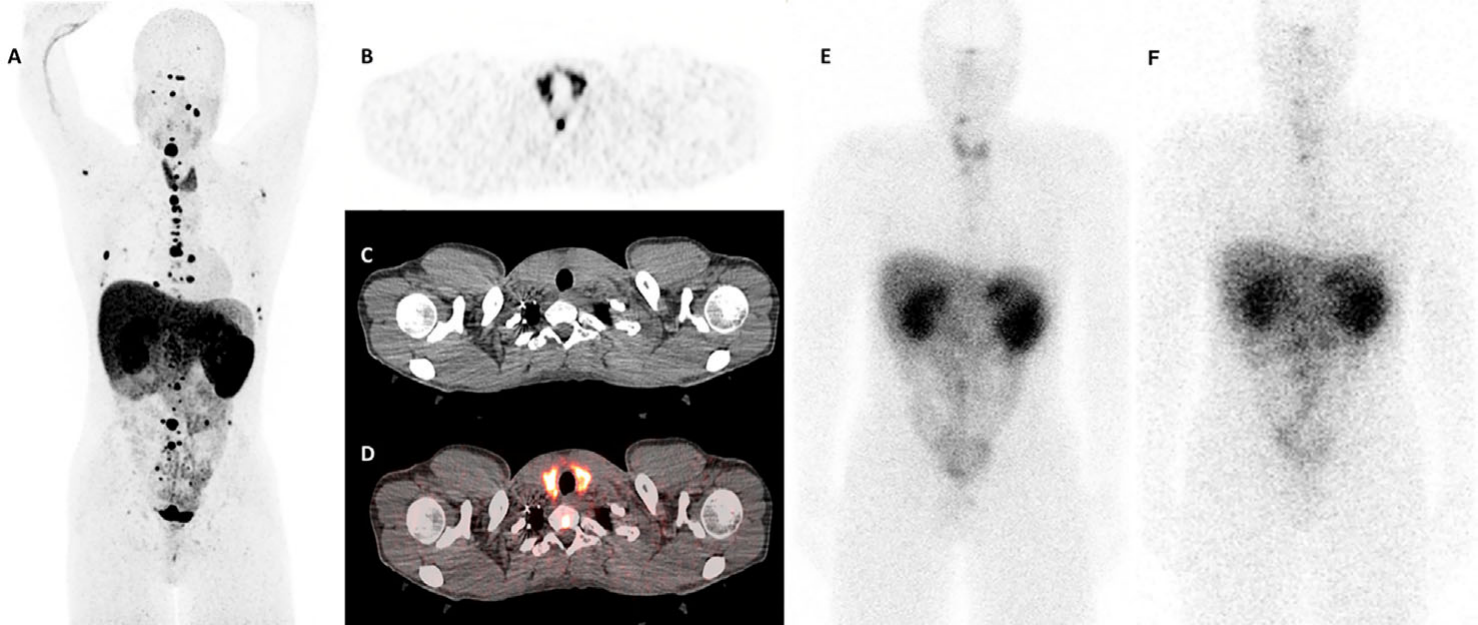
Thyroid uptake patterns in the patient before and after ^177^Lu-DOTATATE therapy. (A) A whole body (maximum intensity projection) view on the diagnostic ^68^Ga-DOTATATE scan demonstrating multiple paragangliomas as well as a diffuse uptake in the thyroid gland with a maximum standardized uptake value (SUVmax) of 14.3. (B–D) Axial slices at the thyroid level: (B) Positron emission tomography (PET) scan only. (C) Computed tomography (CT) scan only. (D) Fused PET and CT images. Images (B, D) demonstrate diffuse uptake in the thyroid gland. (E, F) The 24-h post-treatment whole body single-photon emission computed tomography (SPECT) scans demonstrating diffuse uptake of ^177^Lu-DOTATATE in the thyroid gland (E) After the first cycle of therapy, and (F) after the second cycle of therapy.
